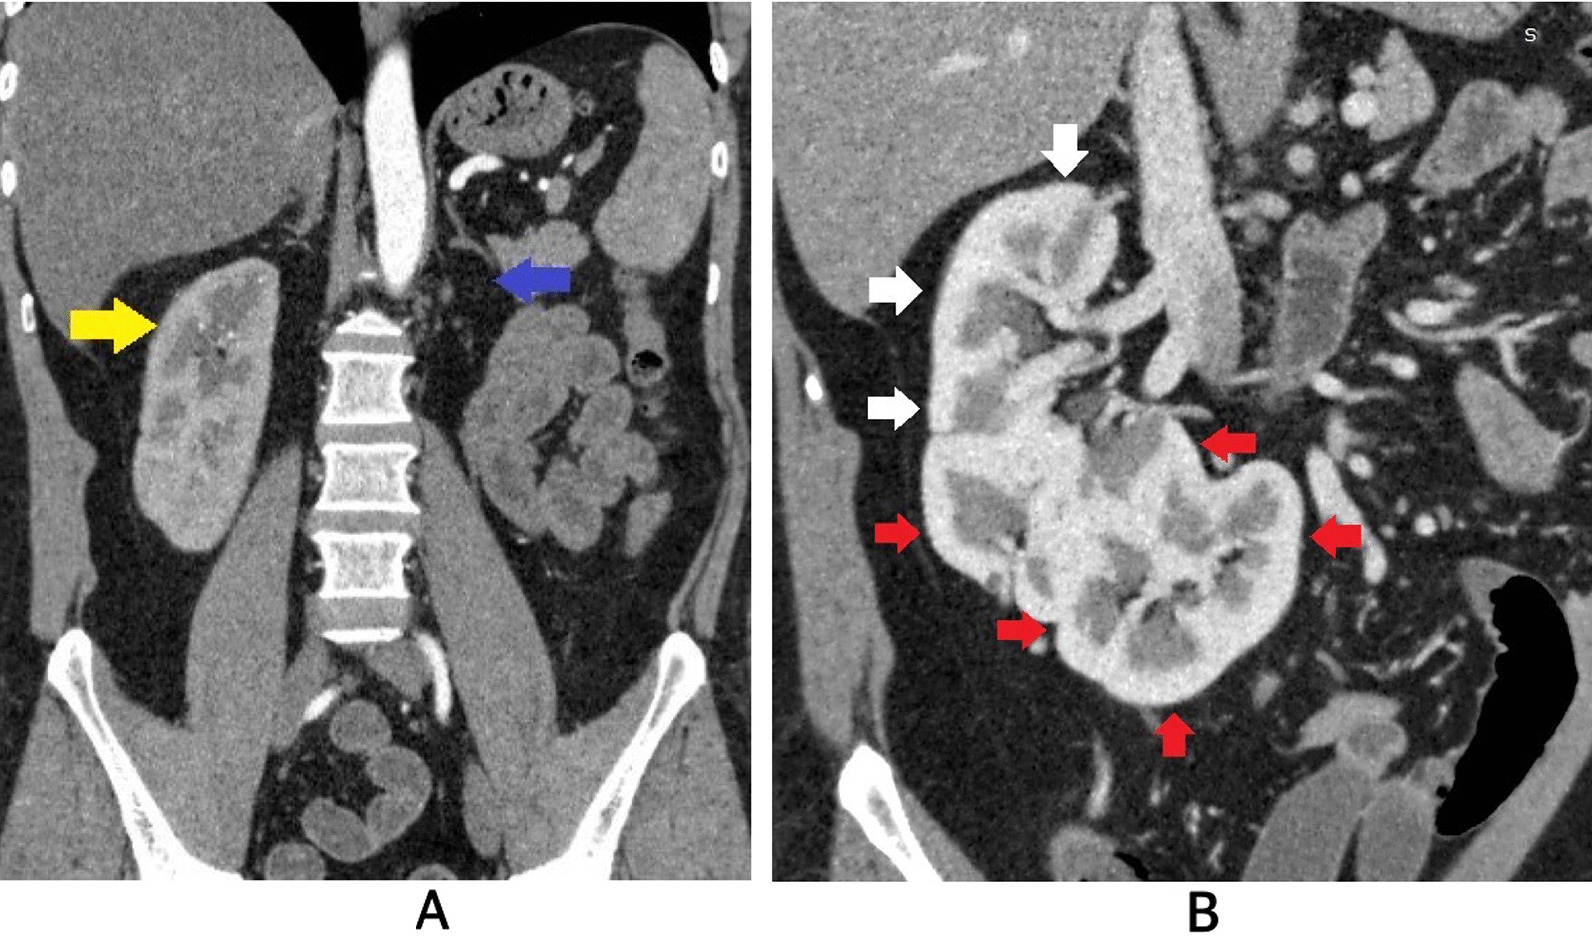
Contrast-enhanced computed tomography images in a soft tissue window. In a, a coronal section image at the level of bilateral renal fossa reveals the absence of the left kidney (purple arrow) and the presence of the right kidney (yellow arrow). b Displays an oblique reformatted image showing the right kidney formed by the fusion of two moieties (each shown by white and red arrows)
